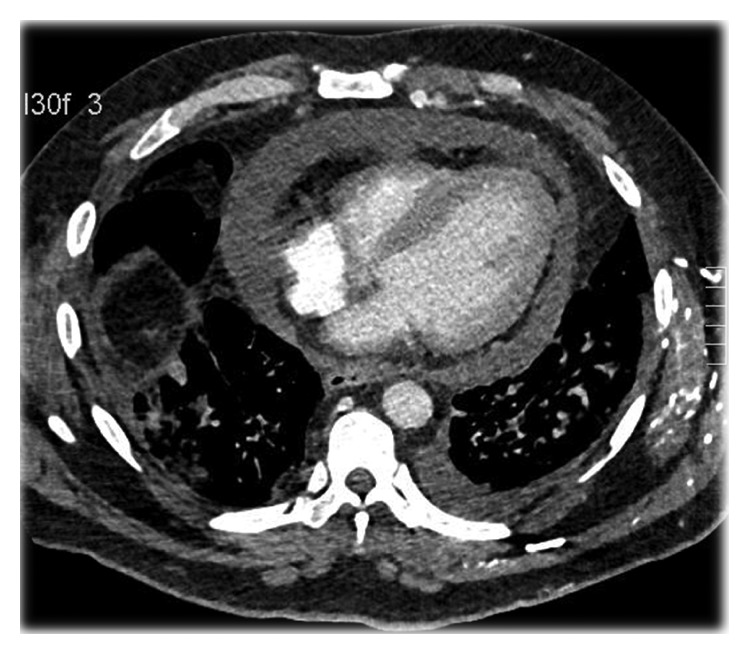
CTA chest with a moderate pericardial effusion.
